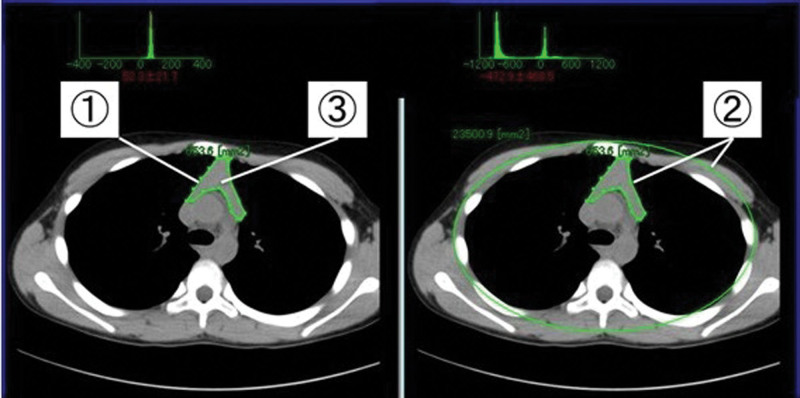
The method of creating ROIs. ROIs were created by tracing the anterior mediastinal tissue using the image reference viewer. The ROIs were evaluated for the area (①) and average CT attenuation value (③). The ratio of the ROI area with the total thoracic area was also calculated using the area of an ellipse drawn around the bilateral ribs, sternum, and vertebrae at the level of the carina to represent the total thoracic area (②).
